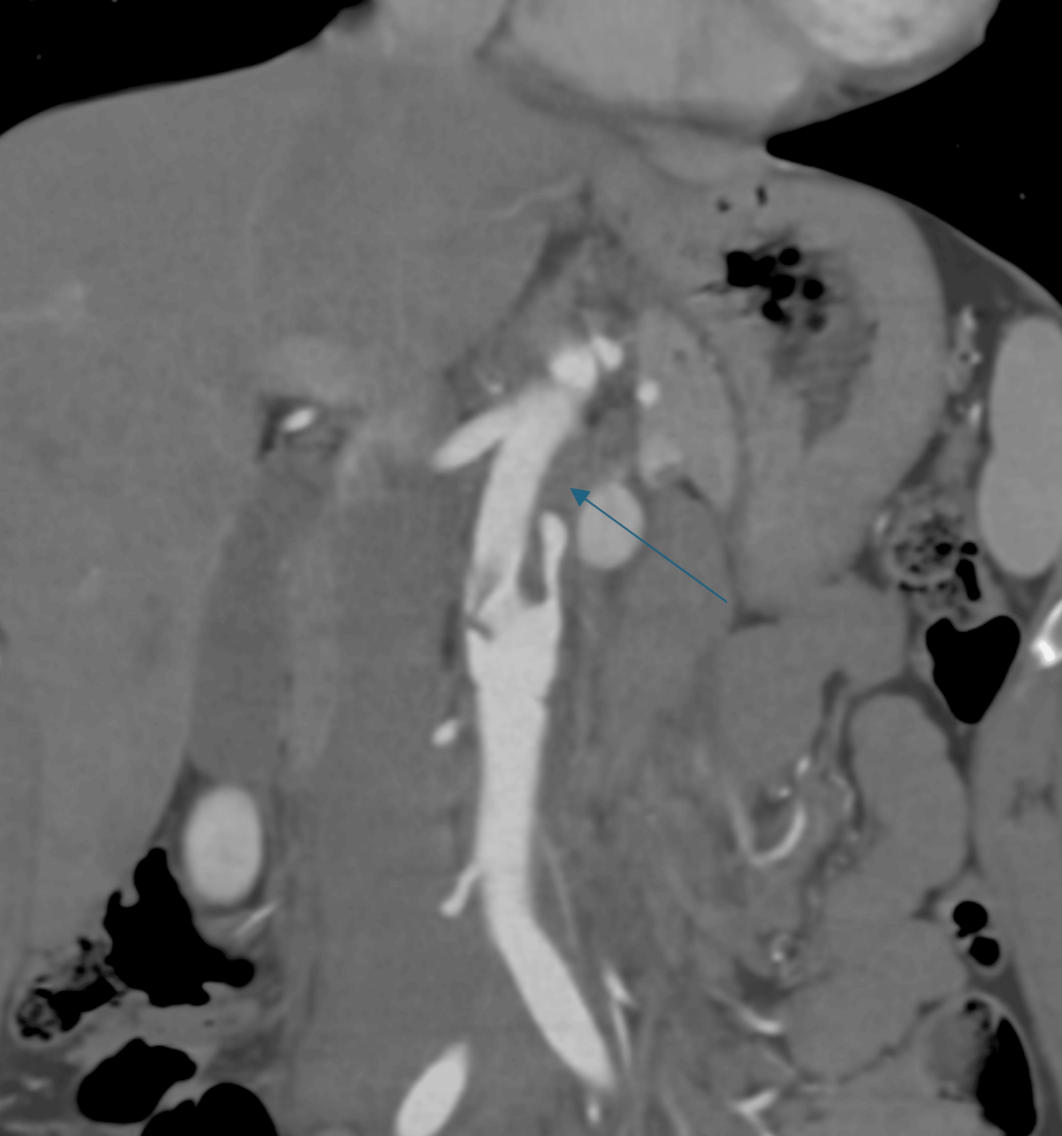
CECT abdomen coronal section showing hypodense filling defect, suggestive of thrombus (shown by thin arrow) within the proximal part of the false lumen CECT: contrast-enhanced computed tomography
